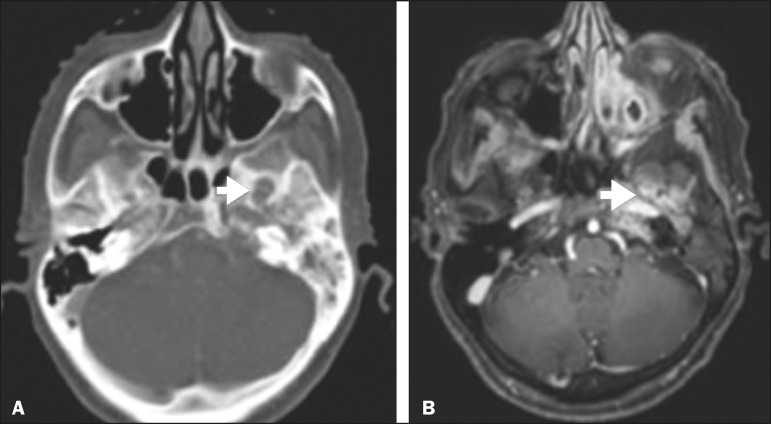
Contrast-enhanced CT (A) and gado­linium contrast-enhanced (B)
